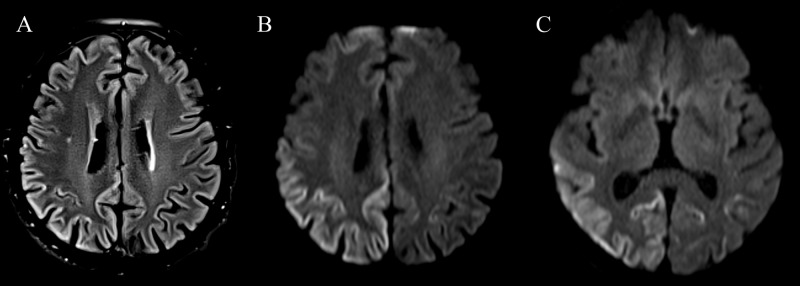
(A) Axial FLAIR and (B,C) axial diffusion-weighted images more inferiorly show persistent cortical FLAIR hyperintensity (arrow in A) and cortical diffusion restriction extending more inferiorly into the posterior temporal and occipital cortex (arrows in B and C). FLAIR, fluid-attenuated inversion recovery
